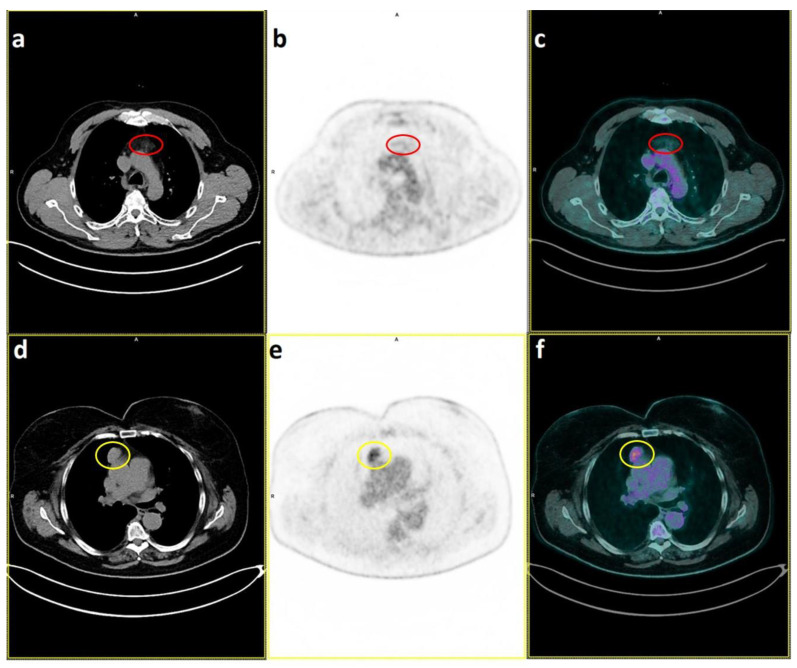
PET CT scan shows absence of uptake in a case of Thymic Hyperplasia ((a–c), with red circle) [18] compared with mild focused uptake in an A-Thymoma ((d–f), yellow circle) [36].
